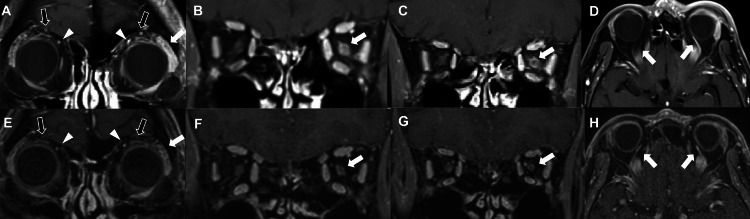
Orbital MRI findings at initial examination and after treatment with 3 courses of steroid pulses (Gd contrast T1-weighted image) Orbital MRI at the initial visit showed the high-intensity lesion of an enlarged lacrimal gland (A, white arrow), and of the mildly dilated superior ophthalmic vein (A. white arrowhead) and supraorbital nerve (A, black arrow) in the left orbital. The optic nerve of the left eye showed a contrast effect, indicating optic neuritis (B, white arrow), which was complicated with a contrast effect around the optic nerve sheath (C, white arrow). Axial section images also showed significant high signal intensity and mild dilation of the superior ophthalmic vein on the left side (D, white arrow). On orbital MRI after treatment, the lacrimal gland enlargement and high-intensity lesion in the left orbit were reduced (E, white arrow), and the left-right difference in the high-intensity lesion of the superior ophthalmic vein (E, white arrowhead) and supraorbital nerve (E, black arrow) was also less noticeable. The contrast effect on the optic nerve and optic nerve sneath also disappeared (F and G, arrowheads).
